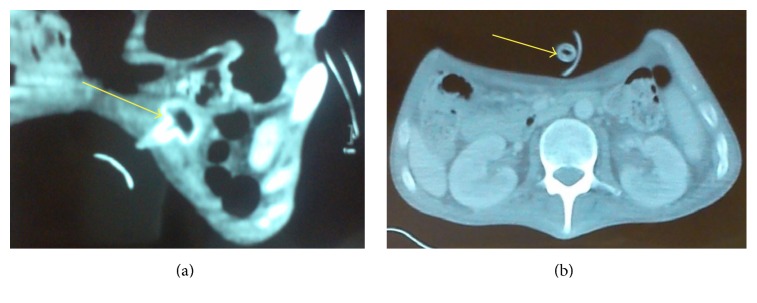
Abdominal computed tomography revealed the internal bumper buried in the gastric wall (yellow arrows) but without subcutaneous tissue or intra-abdominal infection.
